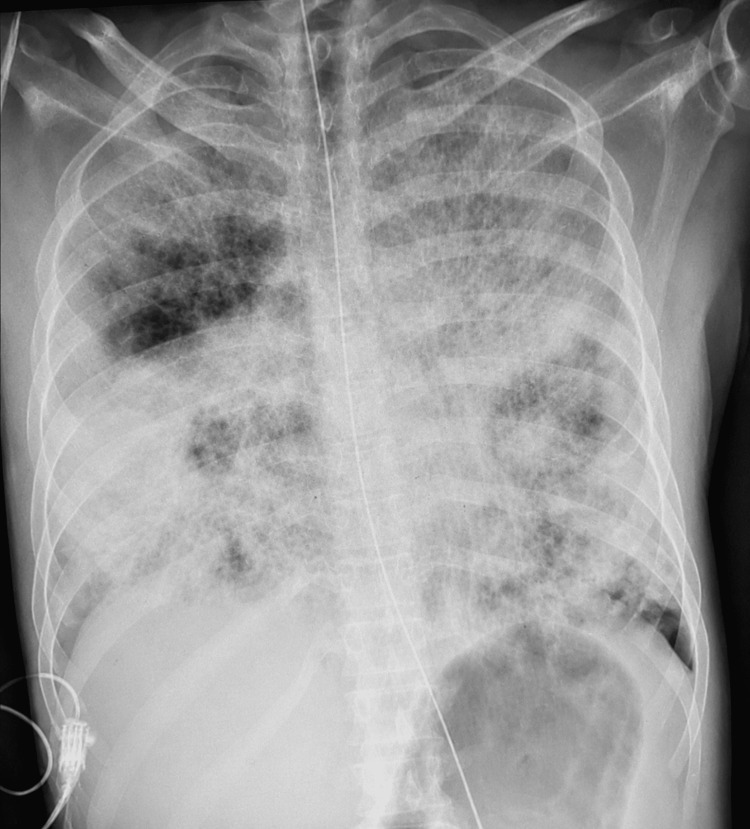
Chest X-ray during deterioration showing bilateral diffuse opacification
